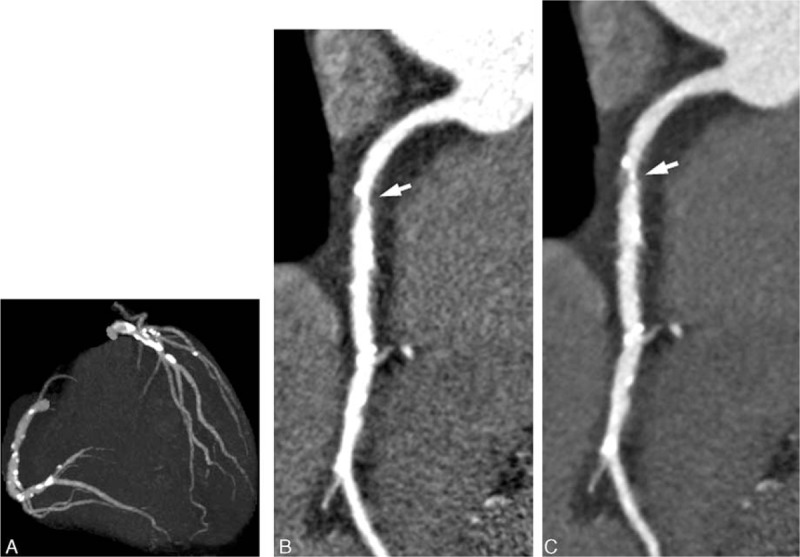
A 73-year-old man with chest pain was suspected of having coronary disease. The patient‘s weight was 56.0 kg at the time of the examination. The i-100-kVp protocol provided remarkable coronary arterial enhancement. The HIR technique decreased the image noise that is seen with the 100-kVp protocol in the maximum intensity projection (MIP) image of coronary CTA (A). Right coronary artery (RCA) stenosis (small arrow) was unclear because of the high image noise and high attenuation of the coronary artery in curved multi-planar reconstructed (MPR) image using the 100-kVp protocol with standard preset (B). By contrast, RCA stenosis (small arrow) in the curved MPR image using the O-100-kVp protocol, was clear. There is lower image noise and appropriate attenuation of RCA in C compared with that in B. CTA = computed tomography angiography, HIR = hybrid iterative reconstruction.
